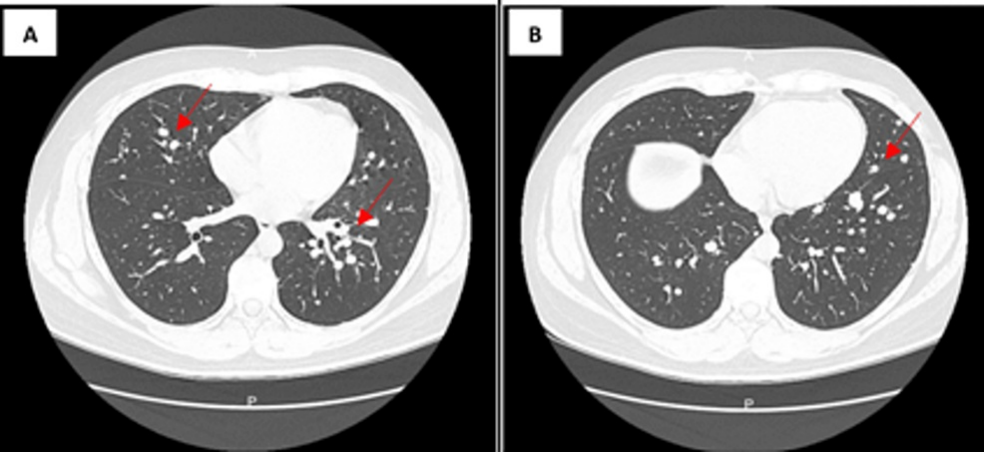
Chest CT demonstrated multiple nodules bilaterally (A) and supradiaphragmatic and paracardiac lymph nodes (B). CT: computed tomography
